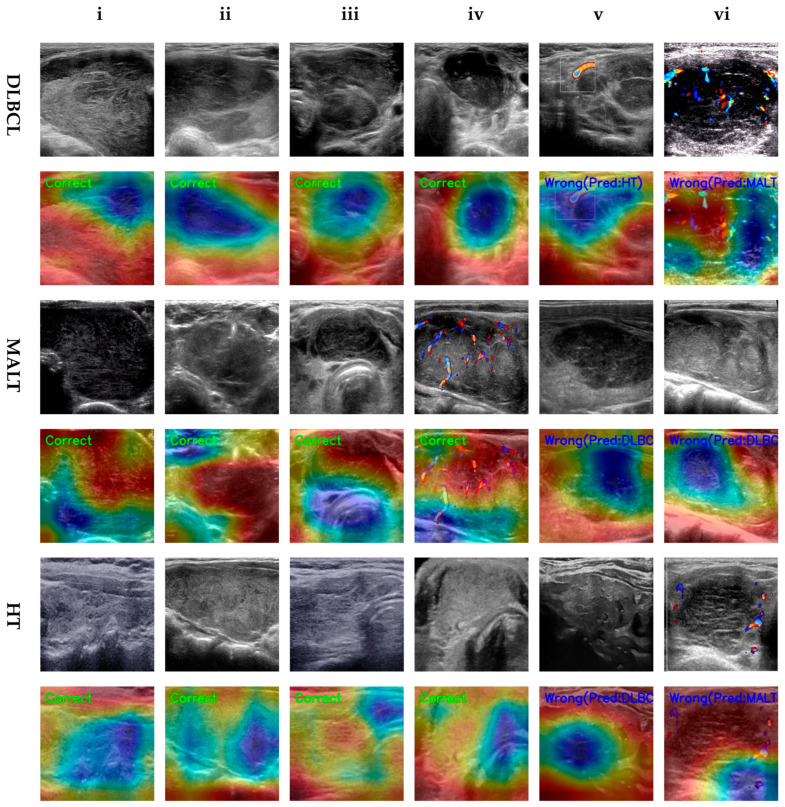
Representative ultrasound images and Grad-CAM heatmaps of DLBCL, HT, and MALT lymphoma. Red regions indicate higher contribution to model prediction, whereas blue regions indicate lower contribution. Columns (i)–(vi) show representative cases. The upper rows display the ultrasound images, and the lower rows show the corresponding Grad-CAM heatmaps. The HT group was characterized by diffuse parenchymal involvement, with or without focal inflammatory hypoechoic areas (also referred to as Hashimoto’s nodules), rather than by isolated tumor-like lesions used for lesion feature analysis.
